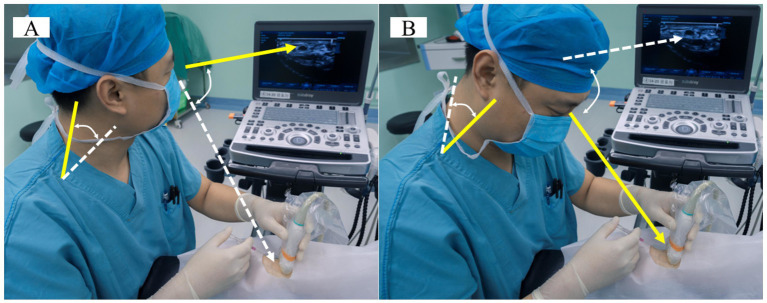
Operator gaze and posture during conventional ultrasound-guided radial artery catheterization. (A) The operator turns the head to view the ultrasound monitor. (B) The operator returns gaze to the puncture site during needle advancement.
